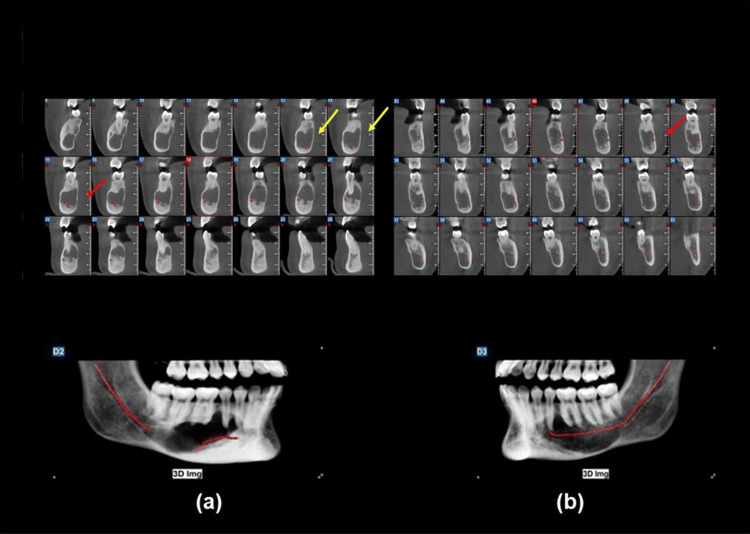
Cone beam computed tomography scan (CBCT) confirmed the presence of two well-corticated and well-defined radiolucent lesions, with a size of 44mm x 13mm bilaterally. A slight buccal-lingual expansion of the cortical plates was observed (red arrows), as well as thinning of the lingual cortical plate. The inferior alveolar nerve had also been displaced (yellow arrows), and no root resorption of the teeth was detected. (a) right side; (b) left side
